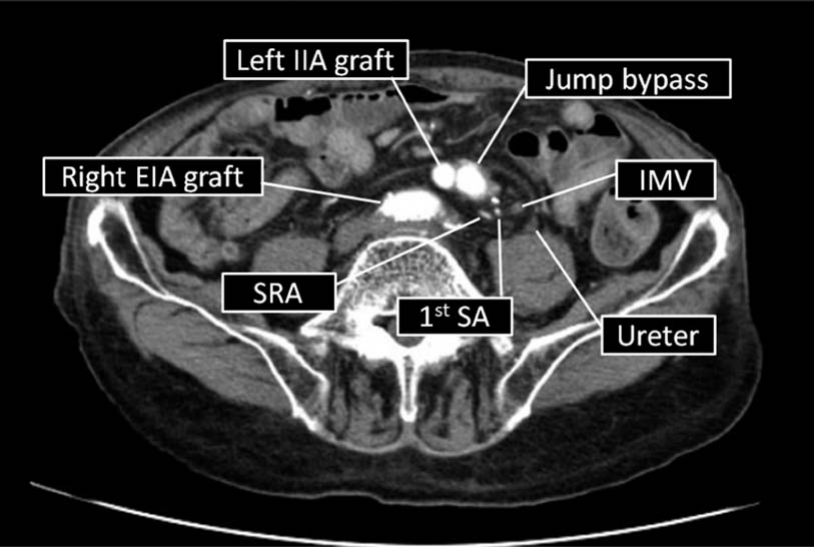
Findings on an arterial phase contrast-enhanced computed tomography image. The image reveals the jump bypass and external iliac artery (EIA) graft on the ventral side of the superior rectal artery (SRA). IIA, internal iliac artery; IMV, inferior mesenteric vein; SA, sigmoid artery.
